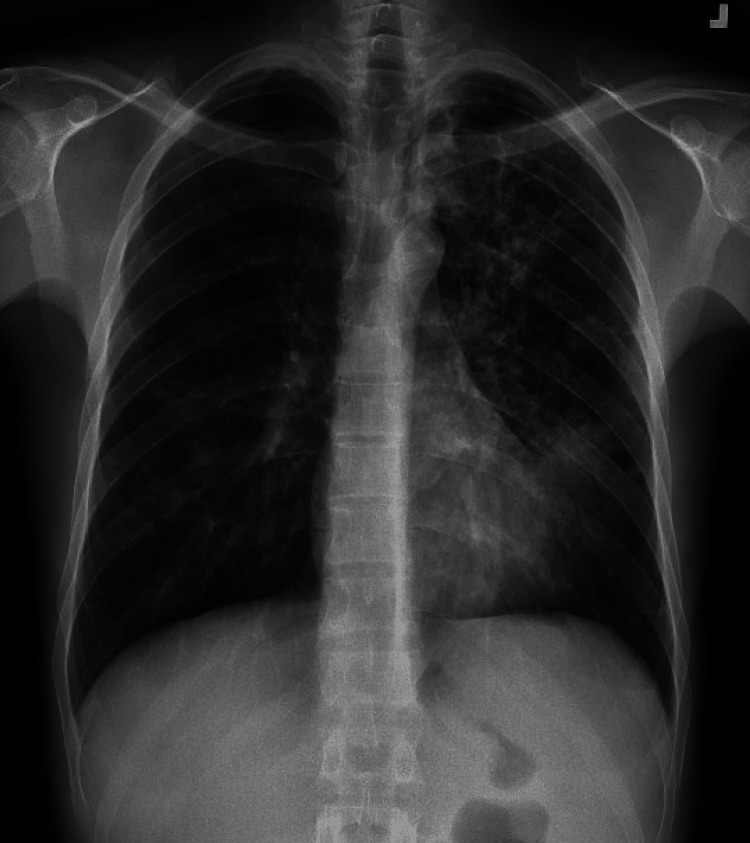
PA view of chest X-Ray PA = posteroanterior
